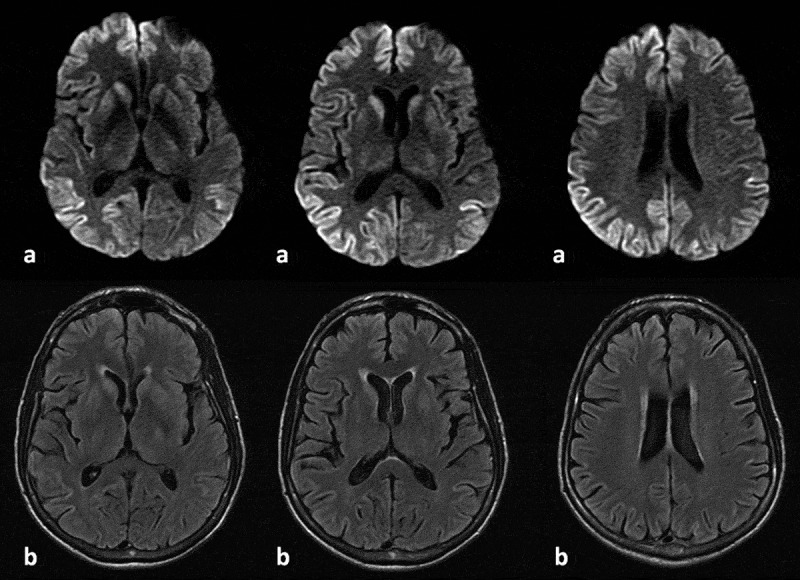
(a) Axial diffusion-weighted image (DWI) sequences show diffusion restriction of water molecules along the bilateral caudate and gyriform cortical-restricted diffusion in the bilateral cerebral hemispheres. (b) Axial fluid-attenuated inversion-recovery (FLAIR) images sequences show discrete areas of hypersignal in bilaterally caudate nuclei, as well as in the cortical regions.
